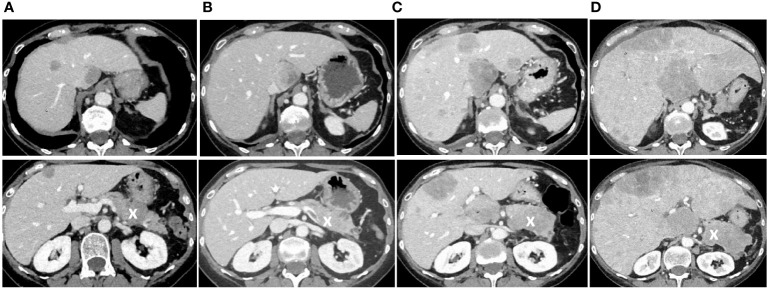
Follow-up CT scans showing tumor progression with hepatic and pancreatic metastases. (A) Follow-up CT scan three months after the end of the initial chemotherapy showing new liver metastases and a new lesion in the pancreas, marked with an X. (B) Follow-up CT scan seven months after the end of initial chemotherapy at the time when increasing doses of tolvaptan were necessary to control hyponatremia. (C) CT scan nine months after the end of chemotherapy at a time when tolvaptan was not effective anymore, and one month later (D) when the patient had beginning signs of liver failure and died shortly after that. In all images, the pancreas is marked with an X.
